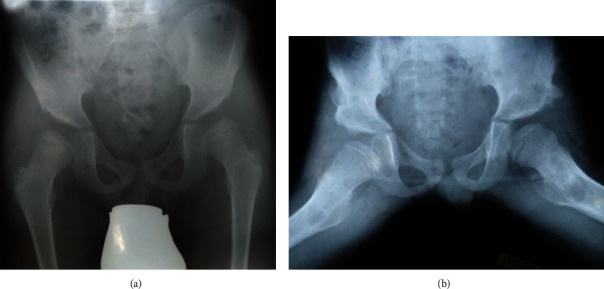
Severe hip dysplasia. Preoperative X-ray images of the patient at 7 years old. Image (a) depicts the pelvis on an anteroposterior X-ray projection. The acetabular angle (AC angle) is 26° and 35° on the left and right, respectively. The lateral center-edge angle (LCE angle) is 14° on the left hip and 8° on the right. Image (b) shows Rippstein's projection of the patient's pelvis.
